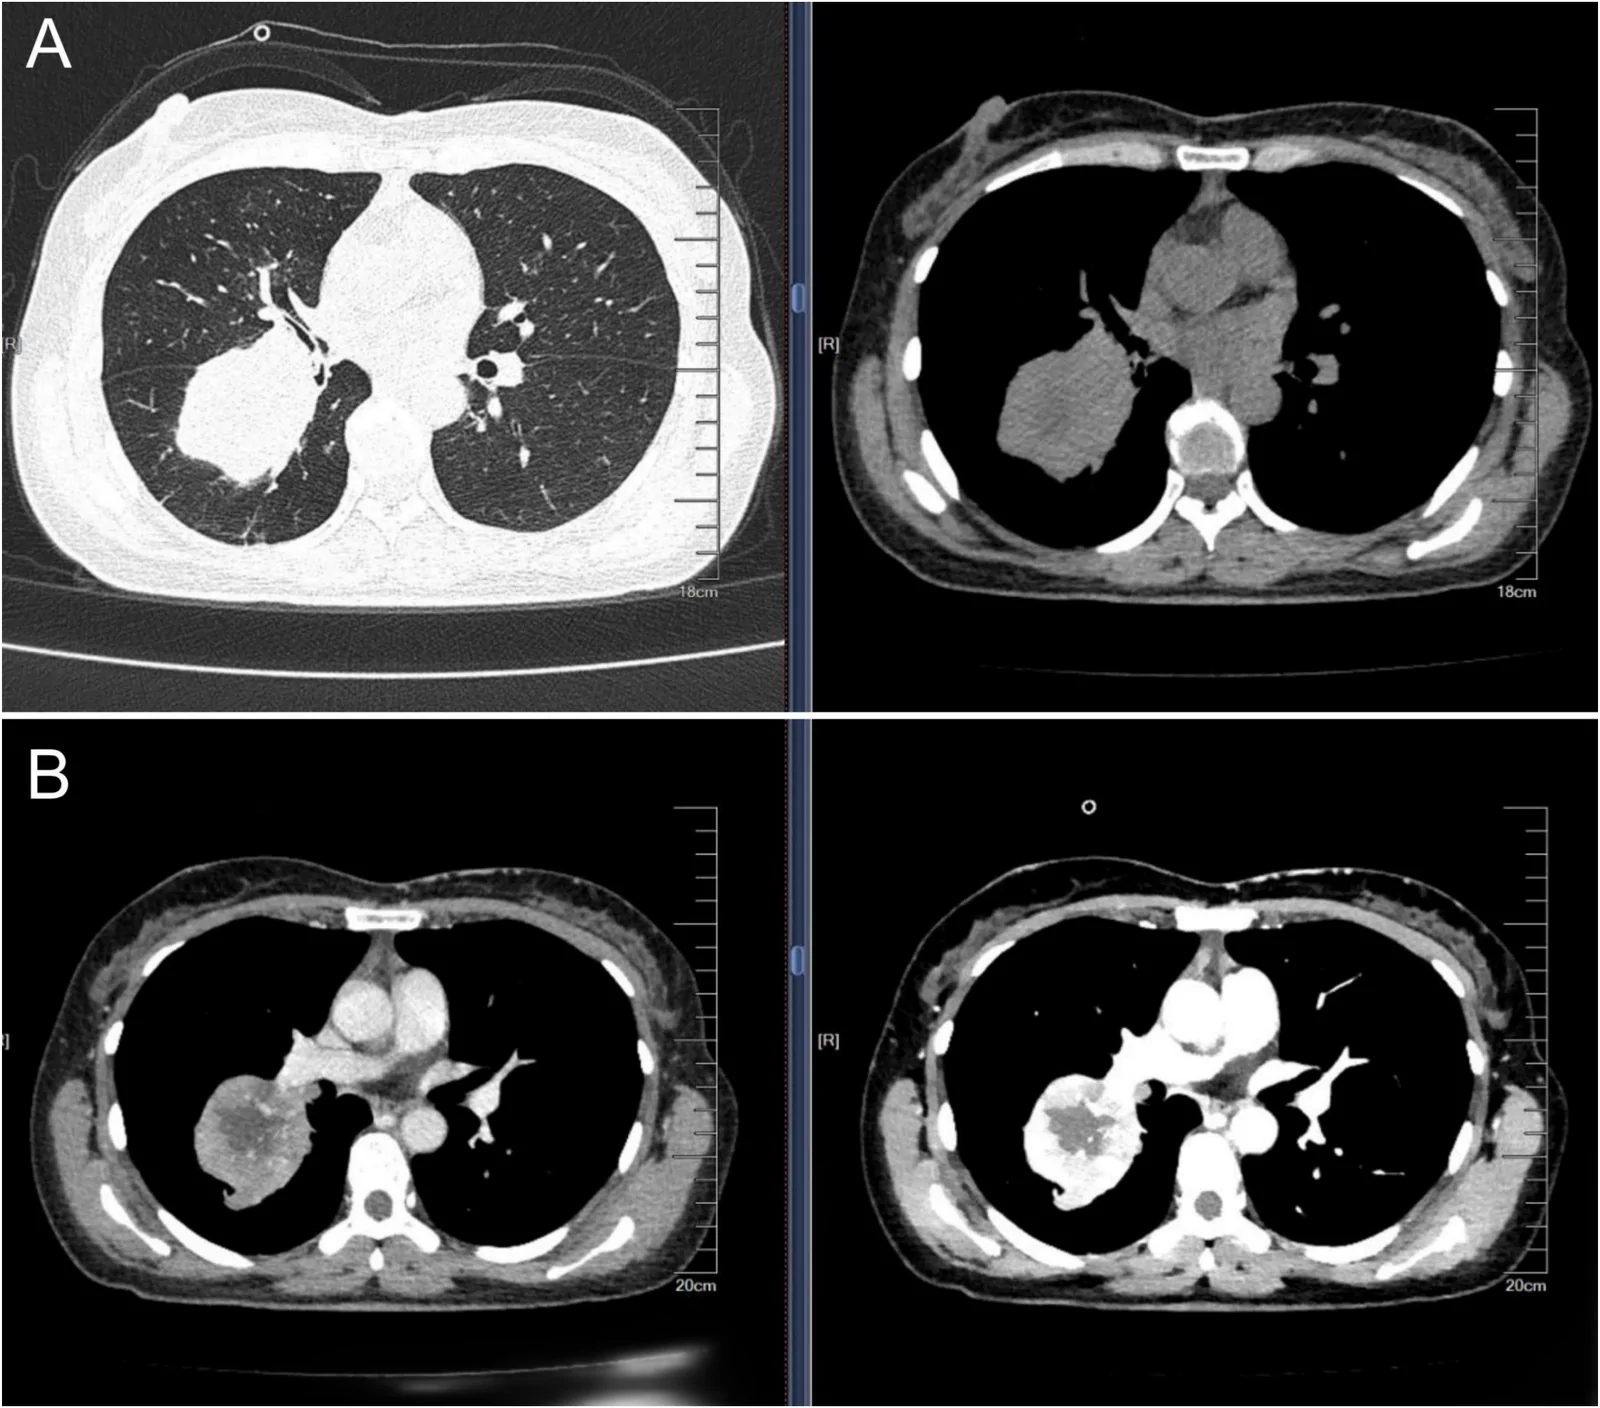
Preoperative axial chest CT series. (A) Non‑contrast axial chest CT (lung window and mediastinal window) showing an approximately 5.5 cm × 4.8 cm heterogeneous soft‑tissue mass with ill‑defined borders adjacent to the right pulmonary hilum. (B) Contrast‑enhanced axial chest CT (mediastinal window) demonstrating peripheral enhancement and central necrosis within the lesion.
